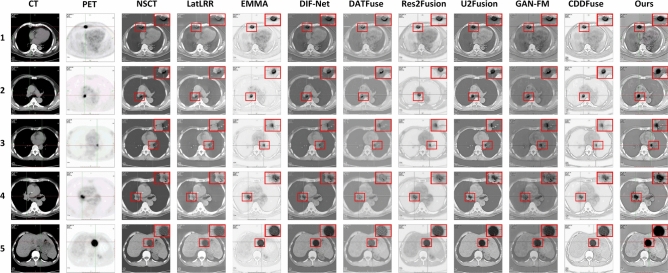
The comparison experiments fusion results of CT mediastinal window images and PET images. Method 1: NSCT; Method 2: LatLRR; Method 3: EMMA; Method 4: DIF-Net; Method 5: DATFuse; Method 6: Res2Fusion; Method 7: U2Fusion; Method 8: GAN-FM; Method 9: CDDFuse; Method 10: Ours.
